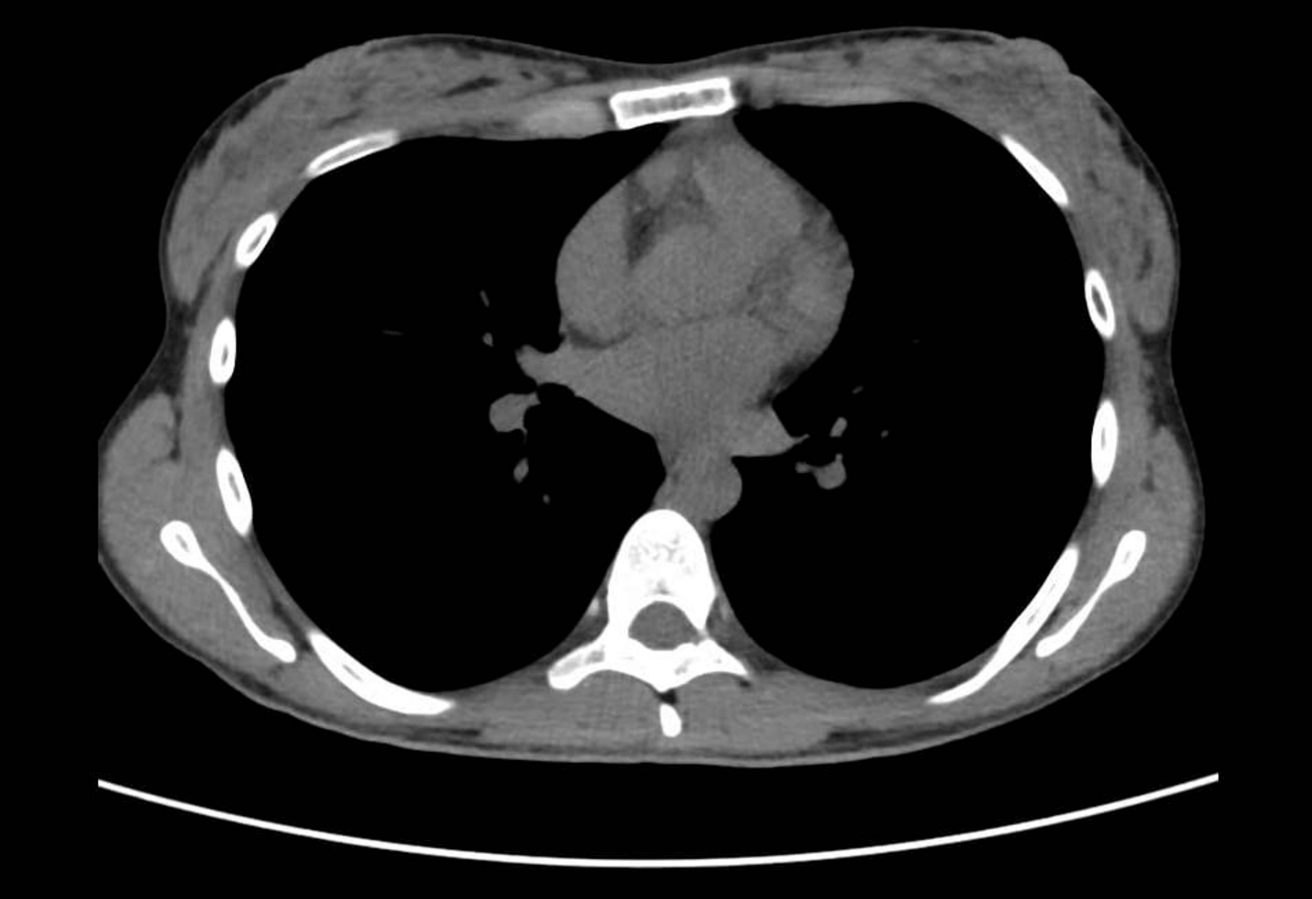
CT chest showing no evidence of mediastinal mass or thymoma.
